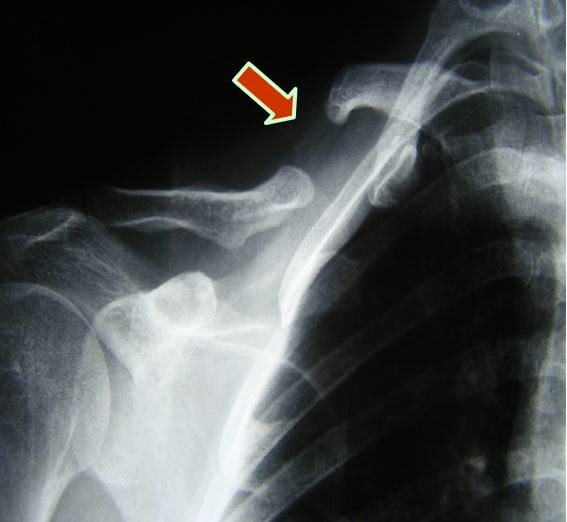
Chest X-ray showing pseudarthrosis of the right clavicle.
